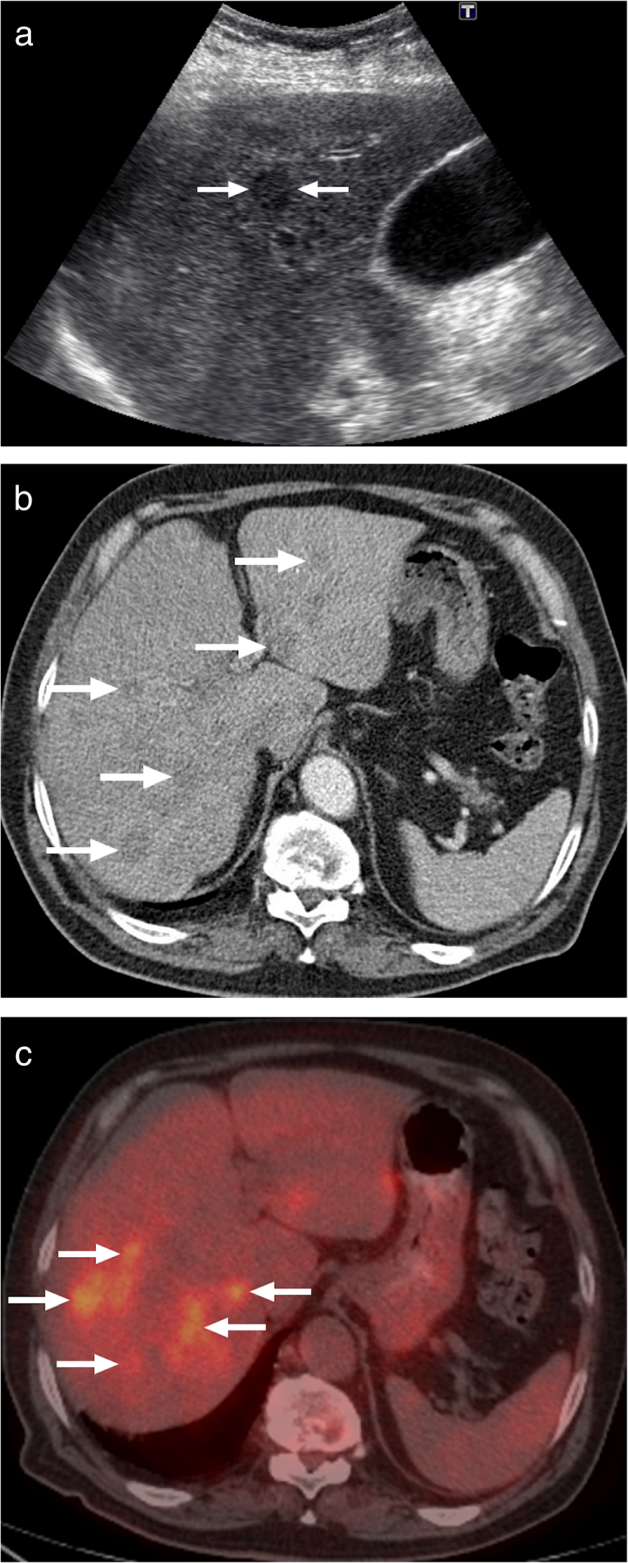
A 60-year-old male with previously treated lymphoma, in complete clinical and imaging remission, now presents with fatigue, fever, and malaise. a Abdominal US image demonstrated several hardly discernible subcentimeter lesions throughout the liver parenchyma. Only one lesion was larger than a centimeter (arrows). b Axial plane postcontrast CT image demonstrates innumerable hypodense lesions (arrows). c On PET-CT, these lesions were found to be metabolically active with intense FDG uptake (arrows). Chest and neck CT studies were unremarkable (not shown). Percutaneous biopsy surprisingly showed noncaseating granulomas consistent with sarcoidosis. Symptoms and imaging findings promptly resolved after corticosteroid treatment
